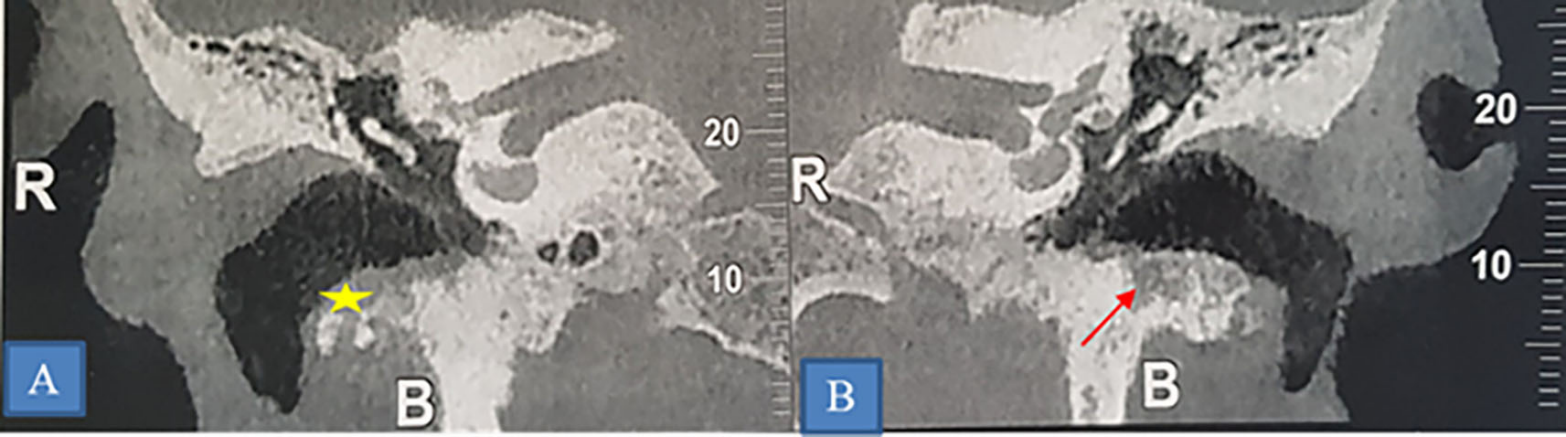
Osteoradionecrosis of the temporal bone. A and B: Coronal section showing of a CT-scan showing bilateral lytic erosion of the tympanic bone (star) and thickening of the walls of the external auditory canal.
